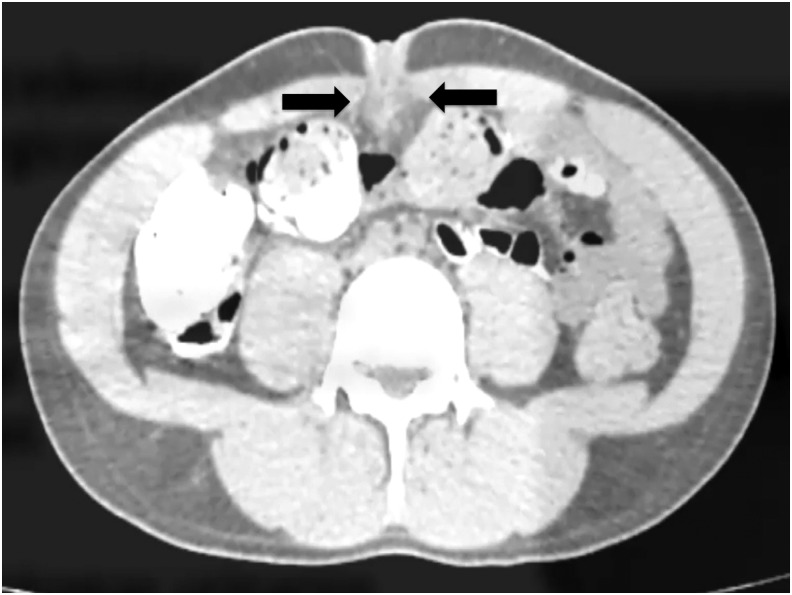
Abdominopelvic CT scan shows a 2.5 × 2.5 midline cystic mass (*arrows*) with inflammatory changes.
